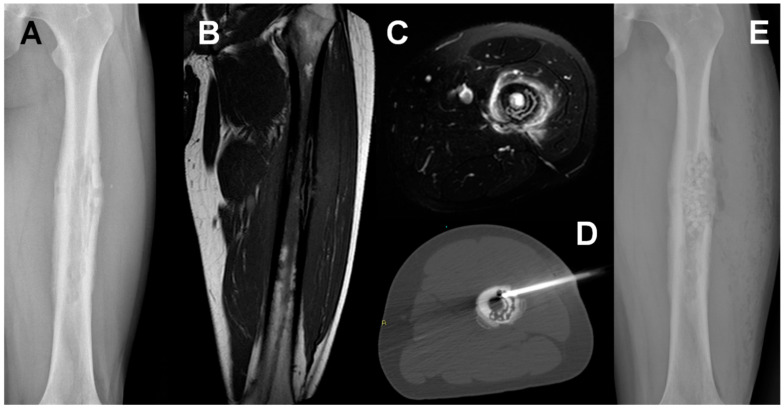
Staphylococcus aureus Osteomyelitis in a 20-year-old man. A conventional radiograph (A). MRI coronal T1w (B) and axial T2w fat-saturated (C) show a permeative lesion of the left femoral shaft. CT-guided biopsy permitted to identify the responsible microorganism (D). Conventional radiograph after surgical treatment showed antibiotic microspheres placed into the bone (E).
